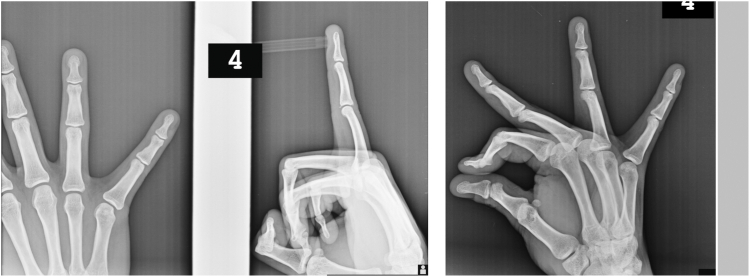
Six-month follow-up radiograph: persistent fracture gap, but full ROM and asymptomatic. ROM, full range of motıon.
